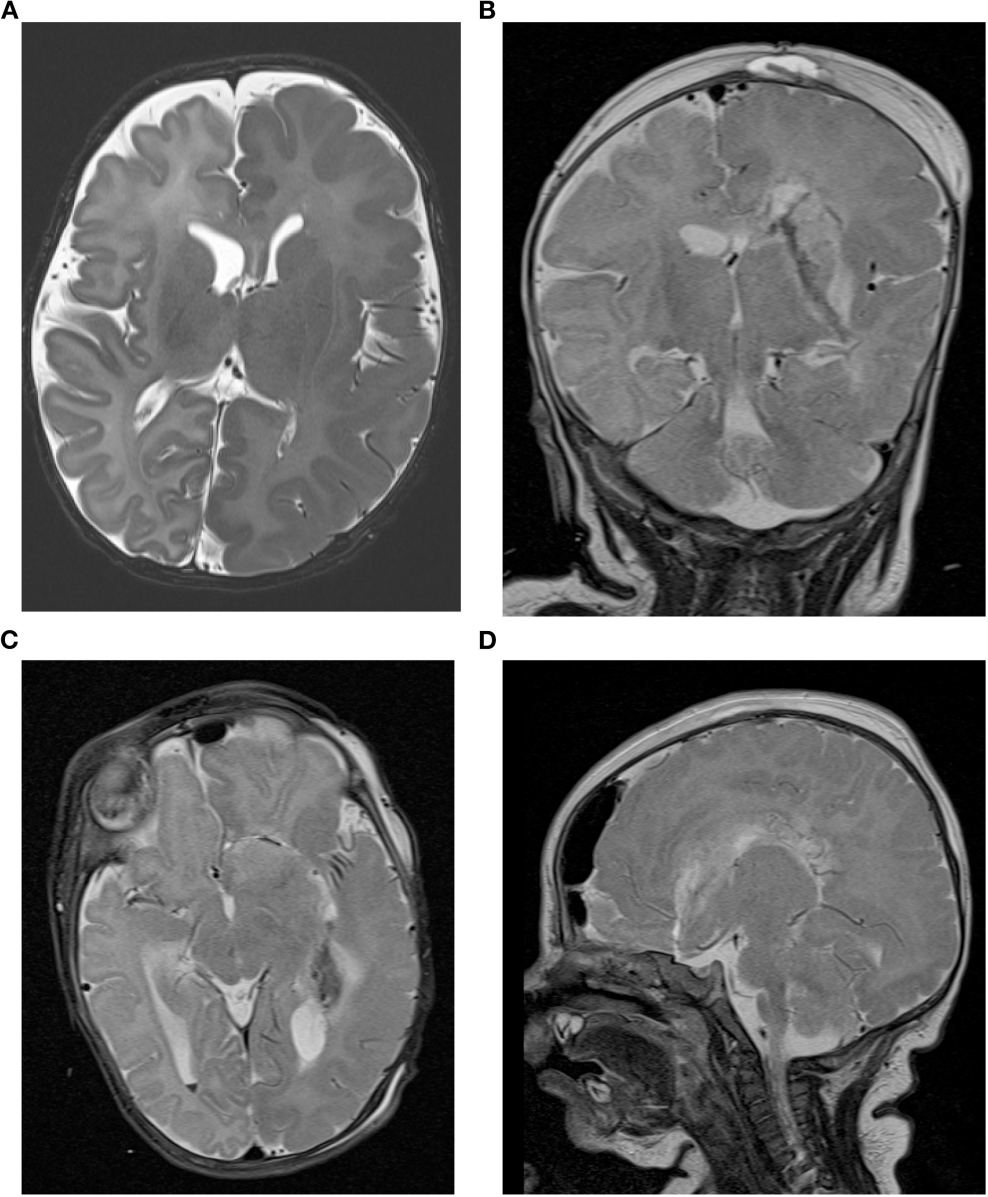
Cranial MRI of the patient. (A) The presurgical axial T2-weighted image shows left hemimegalencephaly with thickened cortex, an irregular gyral pattern and abnormal T2w hypointensity of the white msatter suggestive of accelerated myelination in the entire left hemisphere. Postsurgical coronal (B), axial (C), and sagittal (D) T2-weighted images demonstrate the left sided hemispherotomy.
